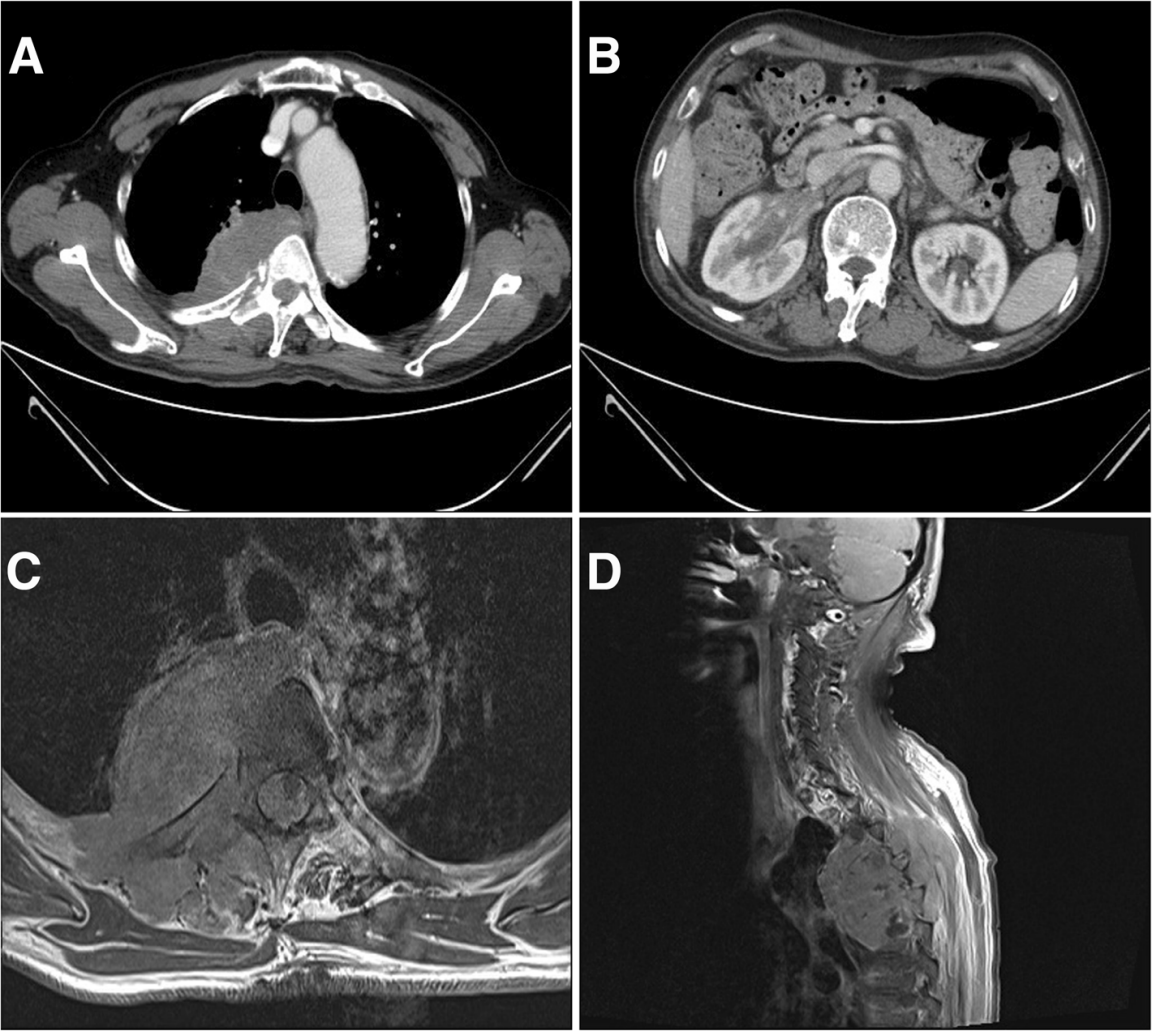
Contrast-enhanced computed tomography and magnetic resonance imaging study at admission. a Contrast-enhanced chest computed tomography shows a large heterogeneous pleural mass involving the right fifth rib and vertebral body. b Contrast-enhanced abdominopelvic computed tomography shows a mass infiltrating the right renal hilum (longest diameter, 44 mm). c Axial T1-weighted magnetic resonance imaging shows a pleural mass with high signal intensity extending to rib, muscle, and vertebral body. d Sagittal T1-weighted magnetic resonance imaging shows a pleural mass with high signal intensity in the right paravertebral area at the level of T3 to T6
